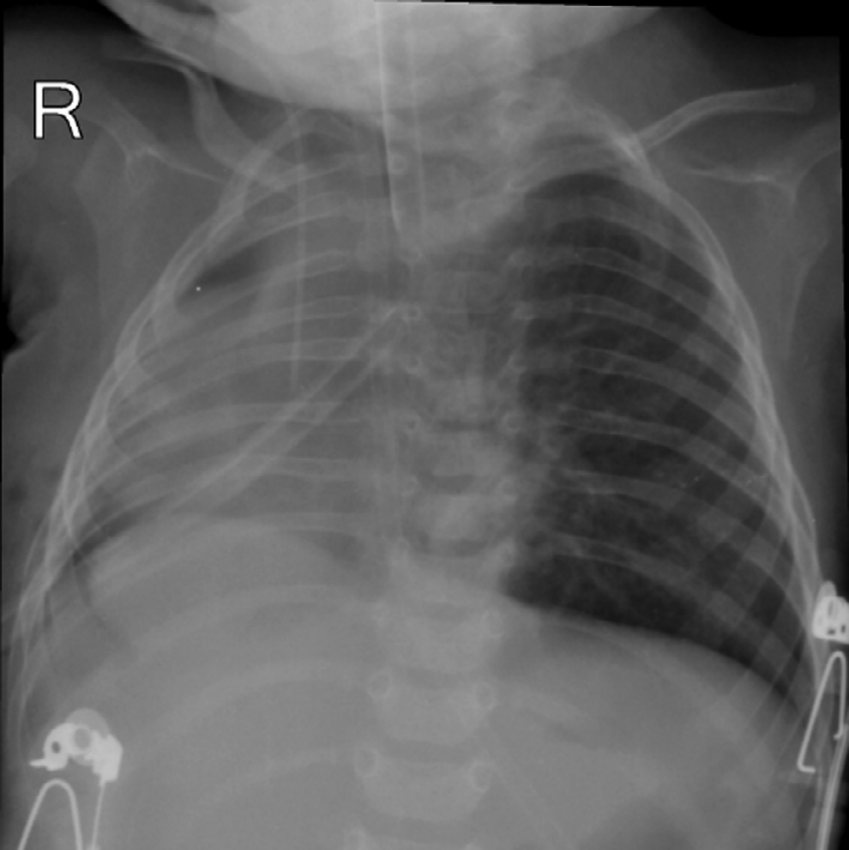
Postoperation chest X-ray shows right pneumonectomy with partial pneumothorax and left side hyperinflation. Also intubation tube and central venous catheter is seen in appropriate positions.
